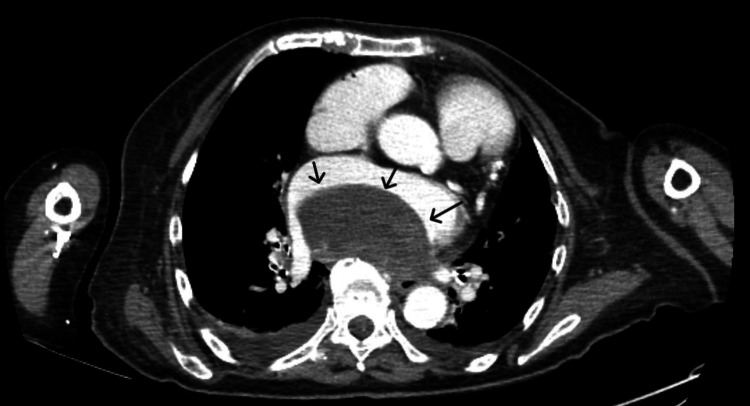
Contrast-enhanced CT image in the transverse section along the atrial plane, revealing a filling defect in the left atrium (indicated by the arrow)
